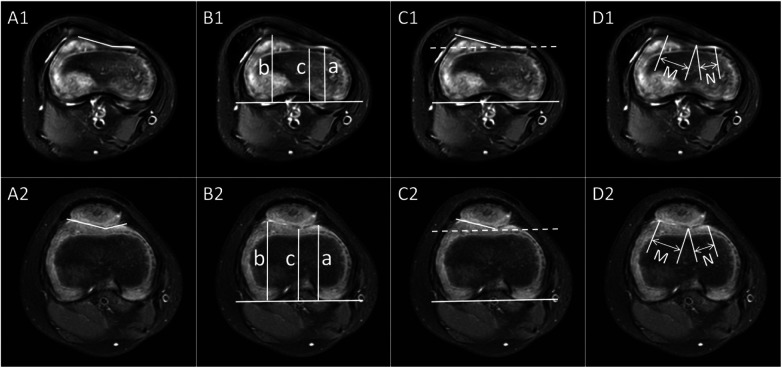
Changes in trochlear morphology following surgical treatment for right HPD in a 6-year-old male patient. (A1–D1) Represent preoperative MRI measurements of the SA, RTD, LTI and TFA, respectively. (A2–D2) Represent MRI measurements of the SA, RTD, LTI and TFA at 26 months postoperatively, respectively.
